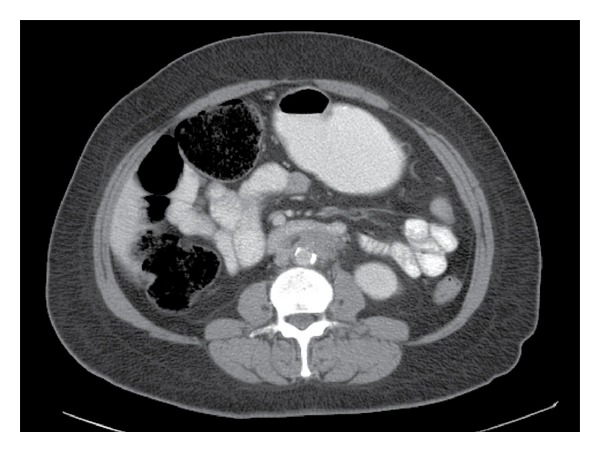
Abdominal computed tomography featuring the aorta with surrounding soft tissue mass.
